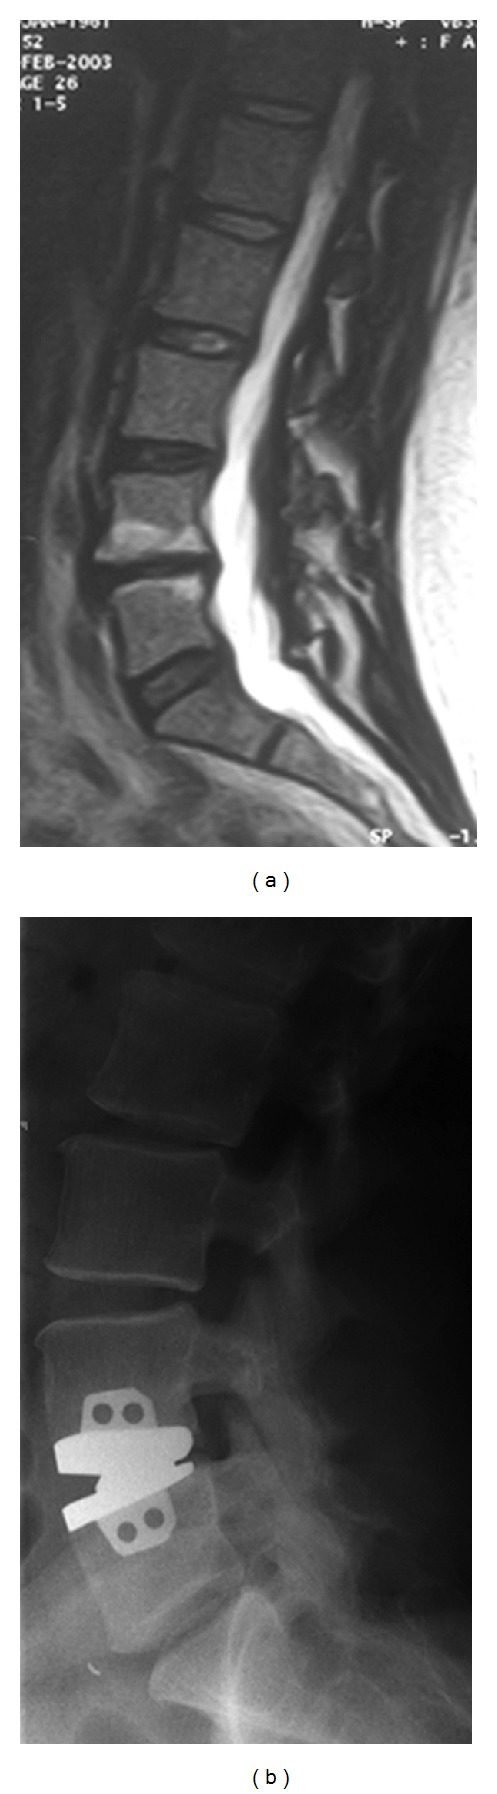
A 30-year-old woman complained of severe back pain attacks. She had no neurological deficits. (a) T2-weighted MRI scans showed advanced degeneration with Modic changes in the L4-L5 disc. (b) Maverick disc prosthesis was applied.
